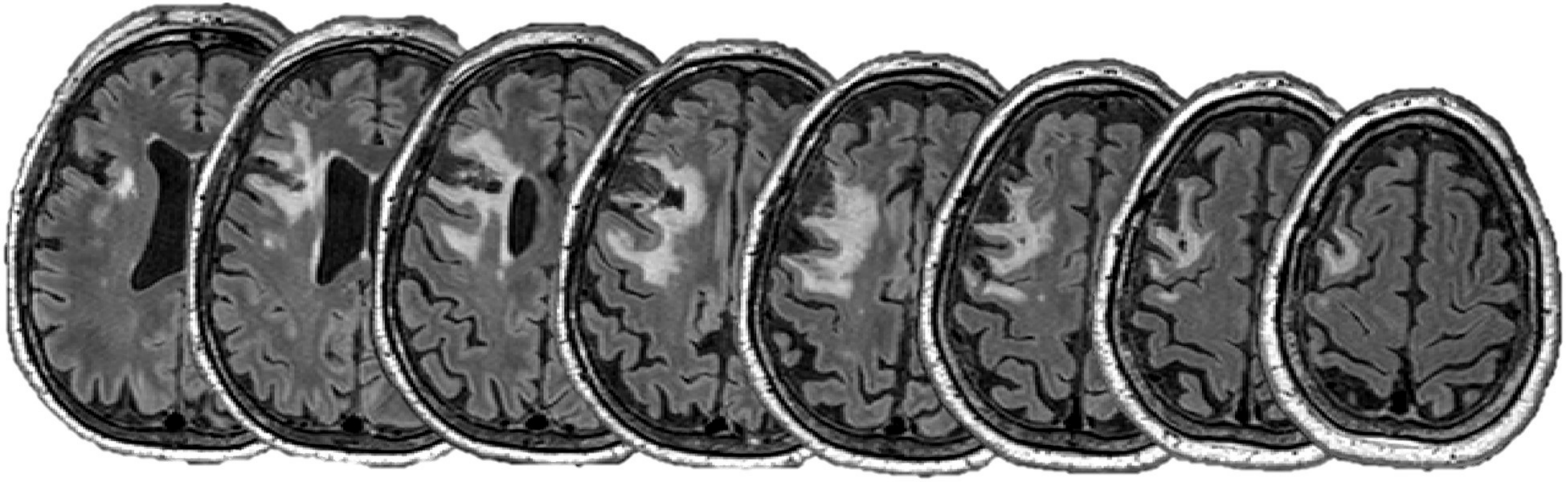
FLAIR images showing the lesion. Images are spaced by 5 mm.
